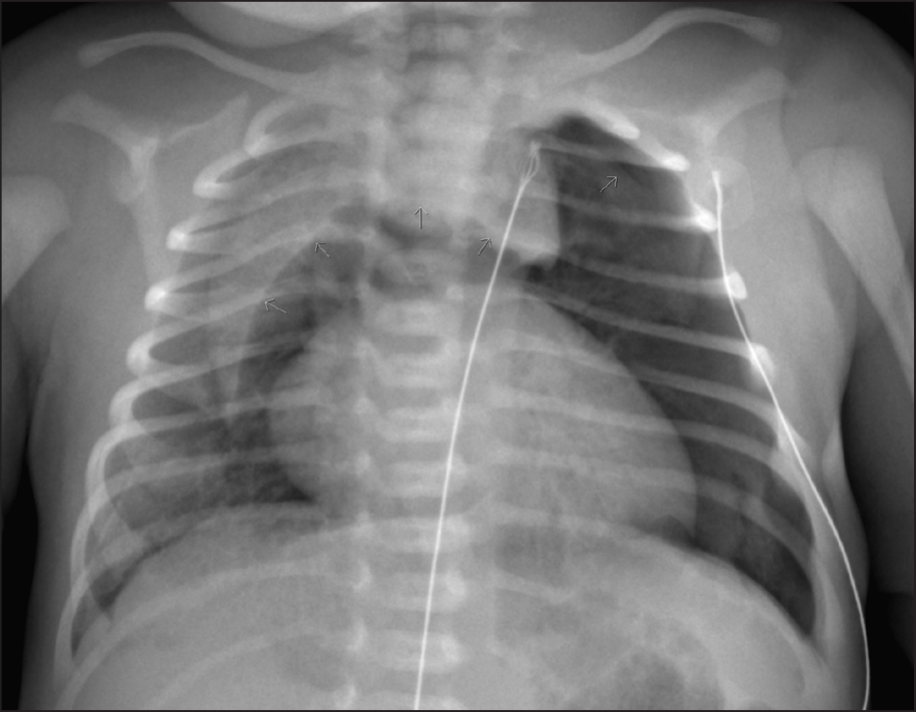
Frontal plain radiograph of the chest, showing the Spinnaker-Sail Sign. Both lobes of the thymus are lifted and displaced into the upper mediastinum, creating a wedge-shaped opacity. This resembles a Spinnaker sail. A pneumothorax can be seen at the left lung apex.
